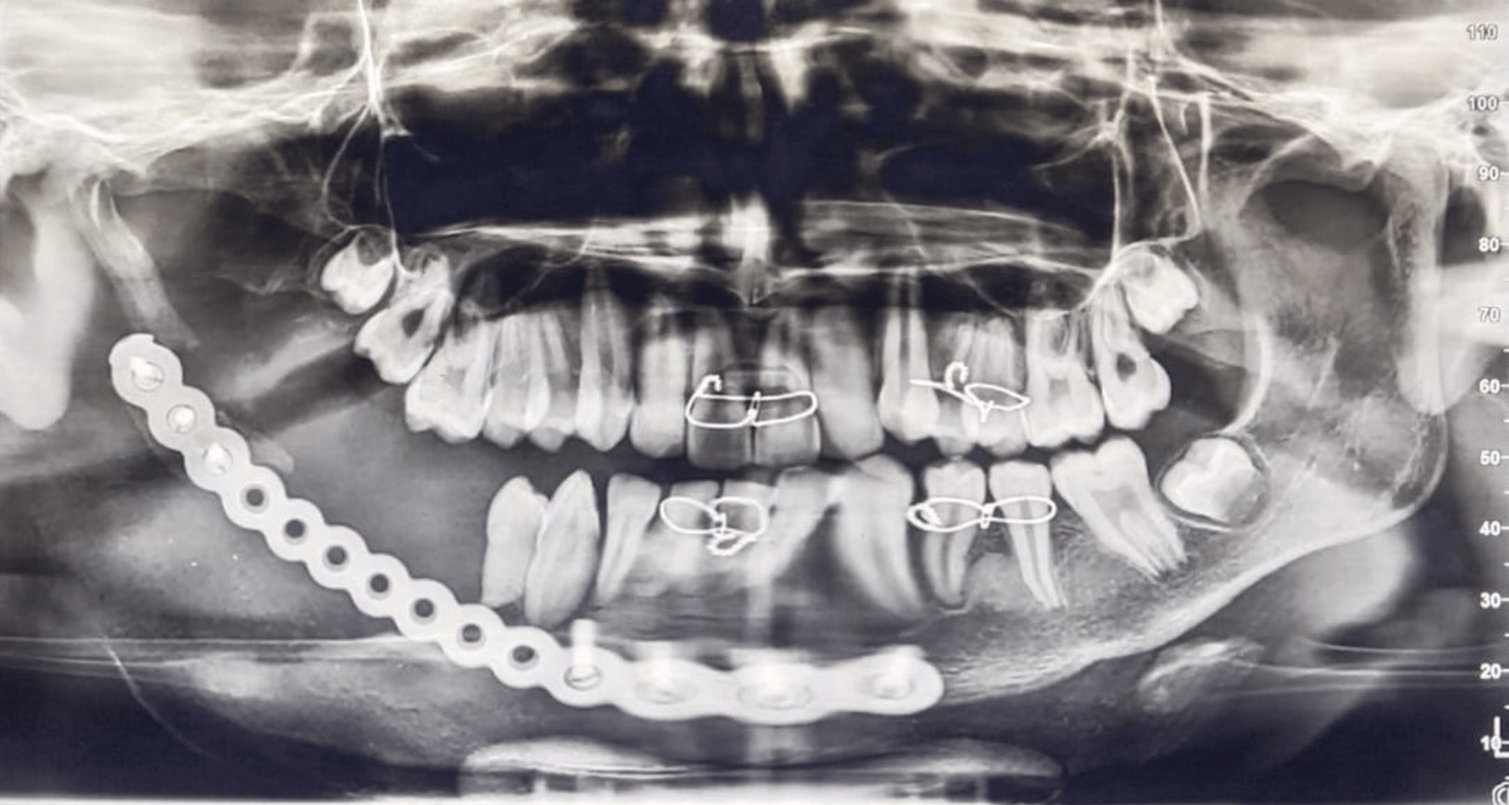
Immediate postoperative OPG. OPG: Orthopantomogram
